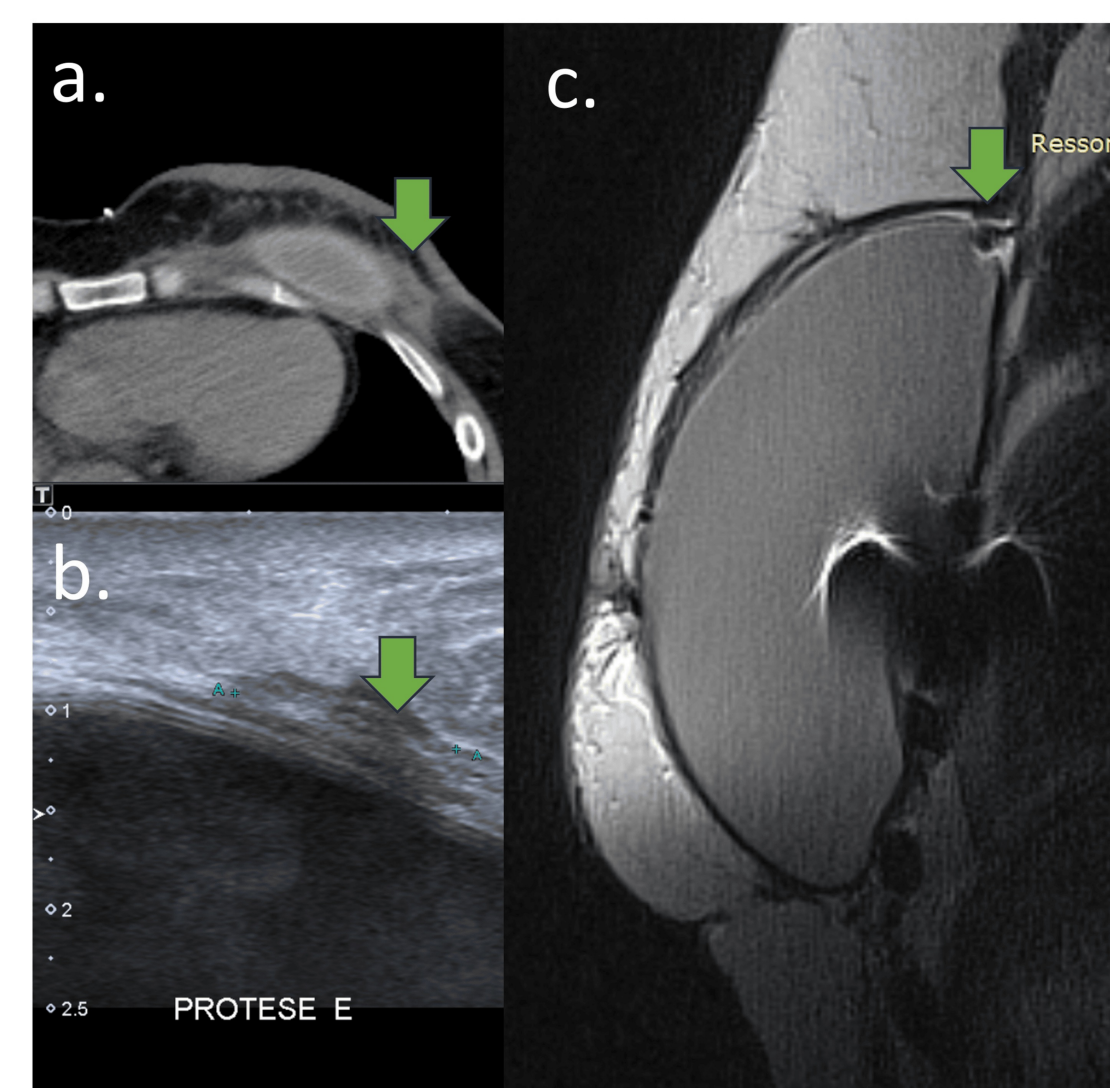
Early complications of acellular dermal matrix (ADM) on MRI A 61-year-old woman with a retropectoral implant and ADM. (a) CT, (b) B-mode USG, and (c) sagittal PD-weighted sequence CT: (a) Intracapsular collection with heterogeneous material and skin thickening (green arrow). USG: (b) Heterogeneous pericapsular tissue with fibrous capsule and implant surface irregularity. MRI: (c) Foldable intracapsular tissue
